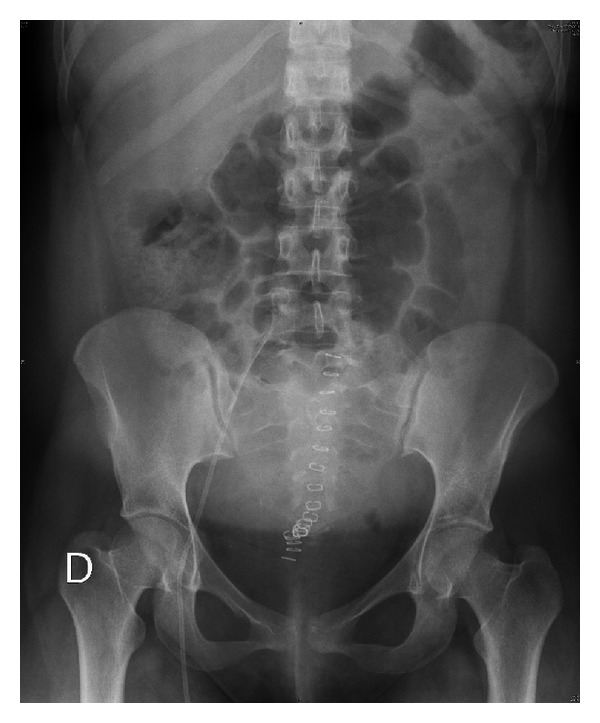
Abdominal X-ray on day three after cesarean section.
